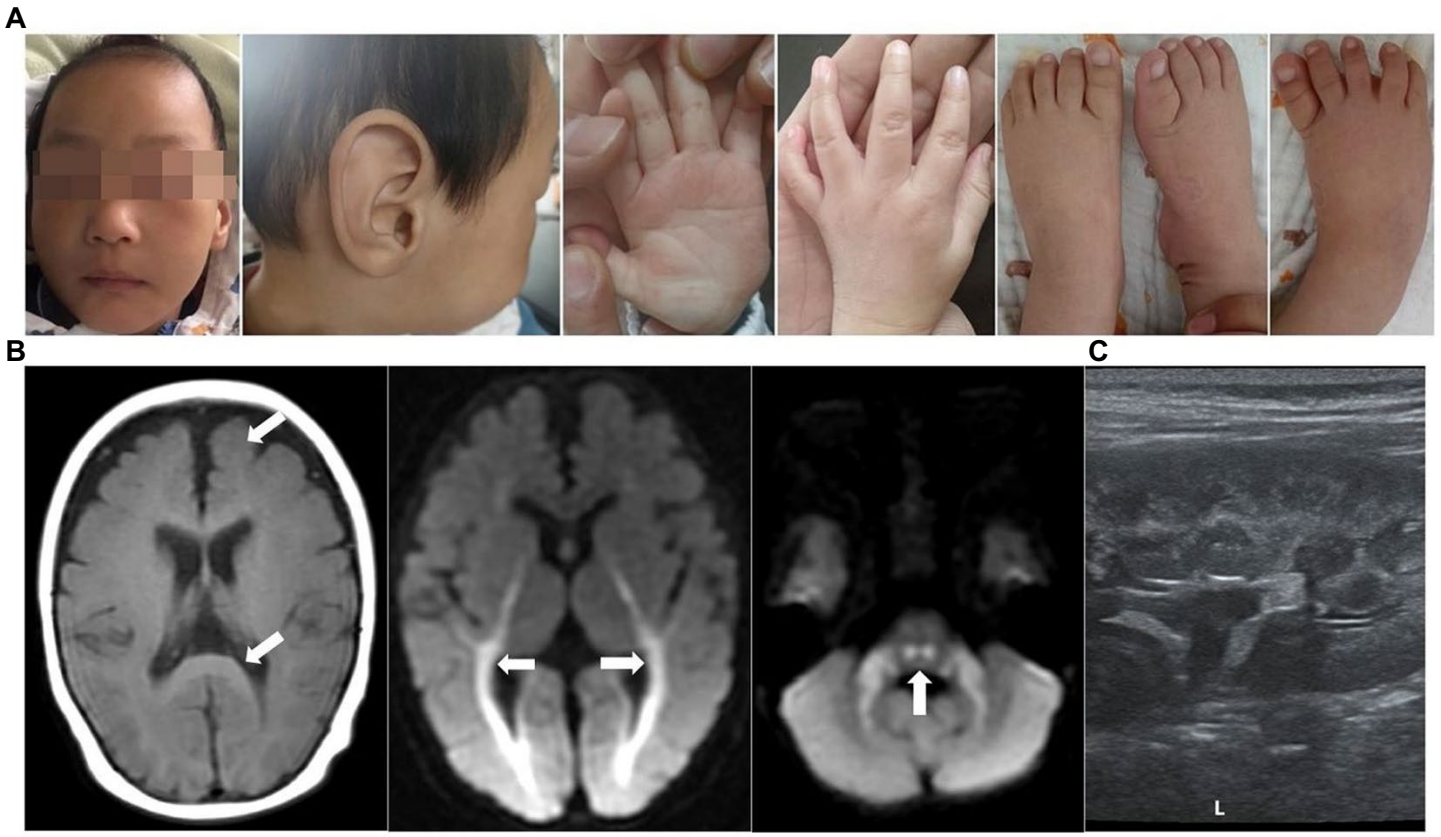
Clinical features of patient 1. (A) Images of faces, hands, and feet showed microcephaly, wide eye spacing, giant ear (large and soft external auricle), disordered palmar pattern, and conical fingers. (B) Brain MRIs performed at the age of 8 months: Axial T1-weighted and DWI images demonstrated an enlarged ventricular system, widened bilateral lateral fissure, anterior longitudinal fissure, and frontotemporal extracerebral space, as well as a slightly wider cerebral gyrus (white arrow). (C) Renal ultrasound disclosed enhanced renal cortex.
